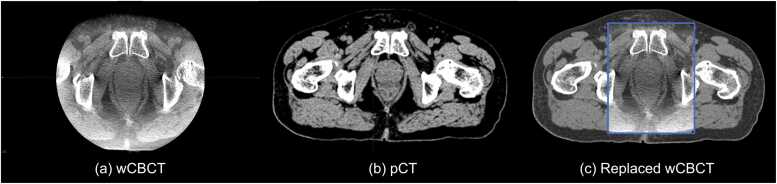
Representative images of the wCBCT (a), pCT (b), and replaced CBCT (c). In panel (c), the region inside the blue line corresponds to the wCBCT, while the region outside the blue line corresponds to the pCT.2.The pCT was deformably registered to each of the 5 replaced wCBCTs for each patient, creating vCTs that reflected the daily anatomical features observed in the wCBCT images.^24^, ^25^, ^26^, ^27^ Contours of the pCT were propagated to the vCTs using deformable image registration (DIR) and, when necessary, manually adjusted by experienced radiation oncologists. DIR accuracy was assessed through visual inspection of anatomical landmarks and contour consistency in all cases.3.To estimate the fractionated delivered dose, the nominal SFUD and IMPT plans were recalculated on each vCT. Positional alignment was assumed using a gold fiducial marker, and forward calculations were performed on each vCT using an in-house dose calculation program based on the same algorithm as VQA.^33^ To validate the consistency between this program and VQA, a 3D gamma analysis was performed to compare the dose distributions calculated by both systems for 3 cases (including both SFUD and IMPT plans). A gamma pass rate of 100% was achieved across all cases under the criteria of 1% and 1 mm, demonstrating excellent agreement between the 2 systems. It should be noted that this result does not necessarily guarantee dose-calculation accuracy.4.Each of the 5 fractionated dose distributions was deformed to the pCT, and the accumulated delivered dose over the treatment period was calculated by summing them on the same pCT using MIM.^34^, ^35^ Consequently, an accumulated dose metric (Metric_accum_) was obtained for each patient for the SFUD and IMPT plans.
